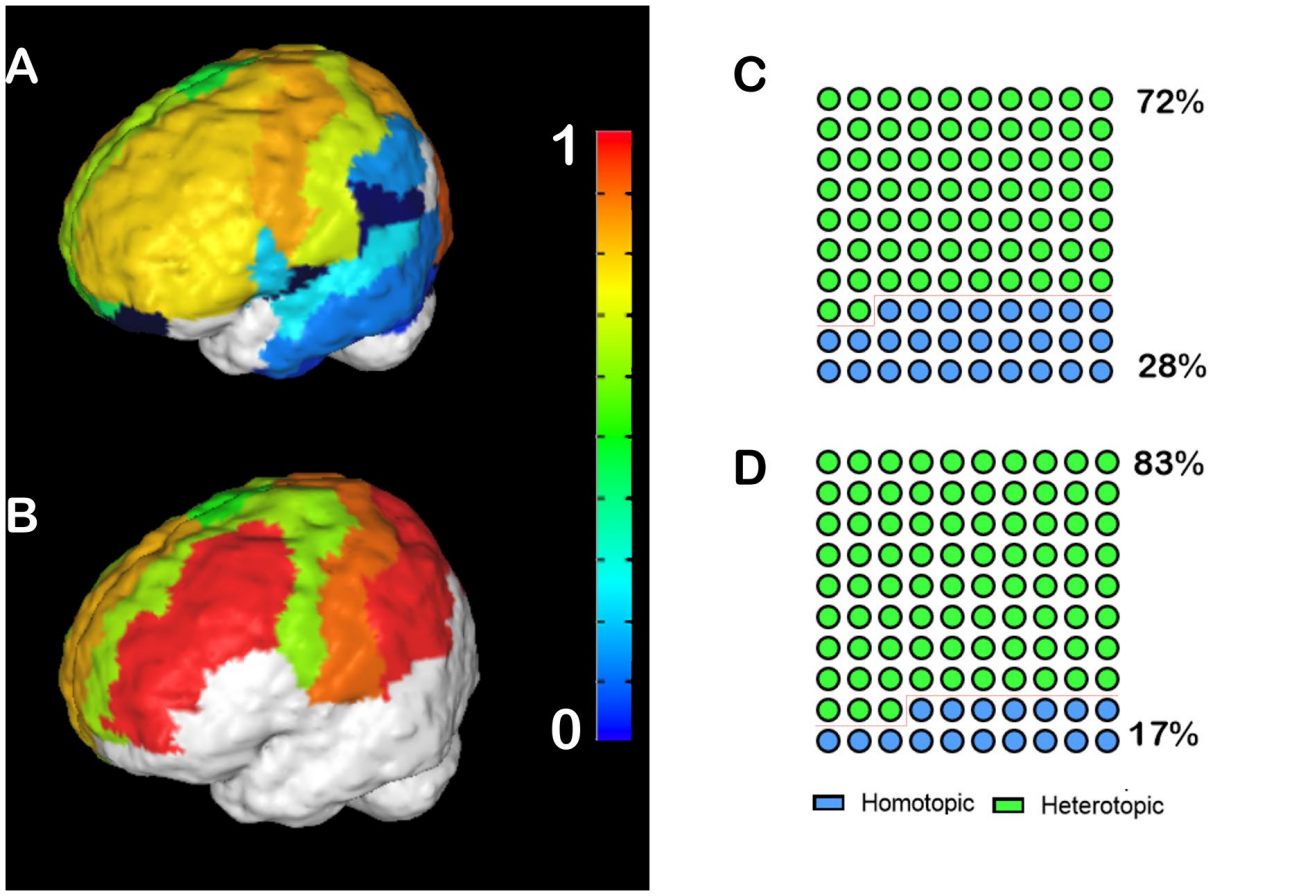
Heterotopicity map of healthy human controls and CCD patients. DWI callosal tractography-based heterotopicity populational average maps of typical subjects (A) and CCD hypoplasic subjects (B). The color bar represents the heterotopicity index scale, with cool colors showing homotopic areas and hot colors showing heterotopic areas. Quantification of the interhemispheric cortical connectivity shows that 72% of typical subjects (C) and 83% of hypoplasic connections are heterotopic (D), revealing a clear impact of callosal dysgenesis on the heterotopic connectivity.
